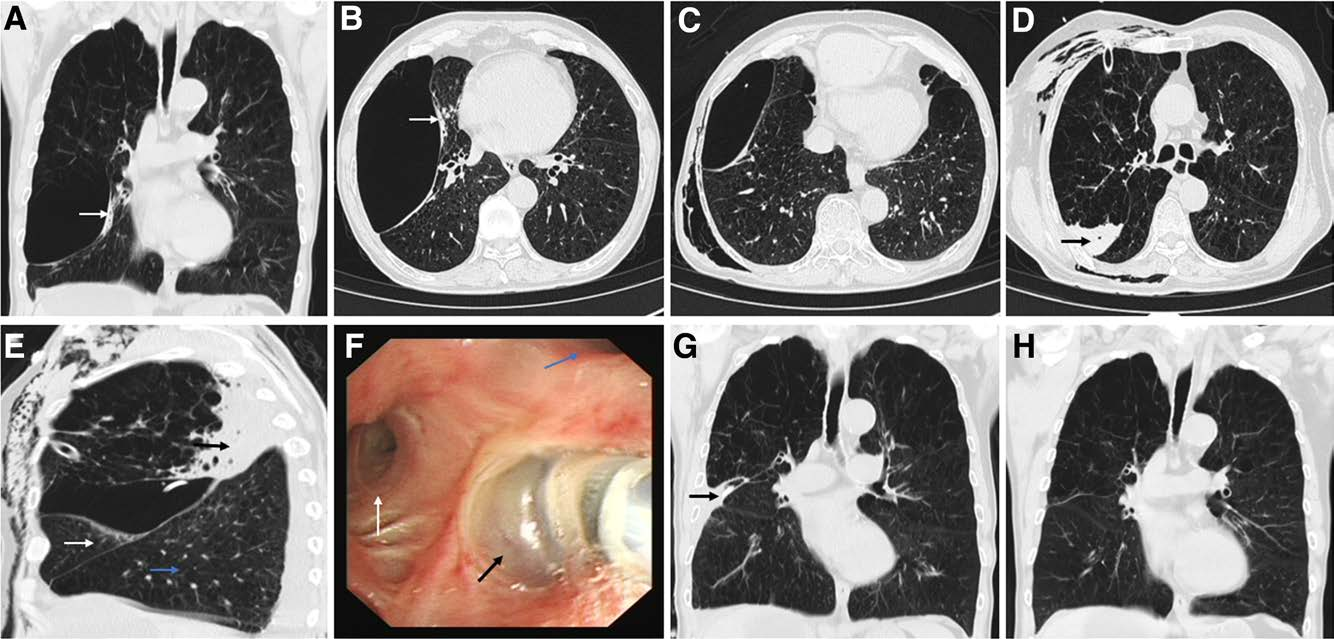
Procedure of GEB volume reduction and confirmation of the GEB target bronchus in the Patient. (A, B) Before treatment, GEB occupied the right middle and right lower lungs, and the radiologist evaluated the target bronchus as the lateral segment of the right middle lobe (white arrow). (C) Under the guidance of CT, a central venous catheter was inserted into the GEB, air was pumped through the drainage tube, and medication was injected. The GEB atrophied to the right upper lobe. (D) Drug deposition in the posterior segment of the right upper lobe (black arrow). (E) Anatomical demarcation reveals: right middle lobe (white arrow), right lower lobe (blue arrow), and posterior segment of right upper lobe (black arrow). Drug deposition and drug-related pneumonia are visible in the posterior segment of the right upper lobe, with reduced GEB and drainage tubes seen in the surrounding area. (F) Bronchoscopic visualization of the right upper lobe bronchial orifice demonstrates: apical segment (blue arrow), anterior segment (white arrow), and posterior segment (black arrow). Using a 3-chamber lithotomy balloon, autologous blood was injected into the posterior segment of the right upper lobe. (G) Before discharge, CT was reexamined, indicating that the GEB was nearly closed (black arrow). (H) No recurrence of pulmonary bullae was observed during a follow-up examination 6 months after the procedure. CT = computed tomography, GEB = giant emphysematous bullae.
